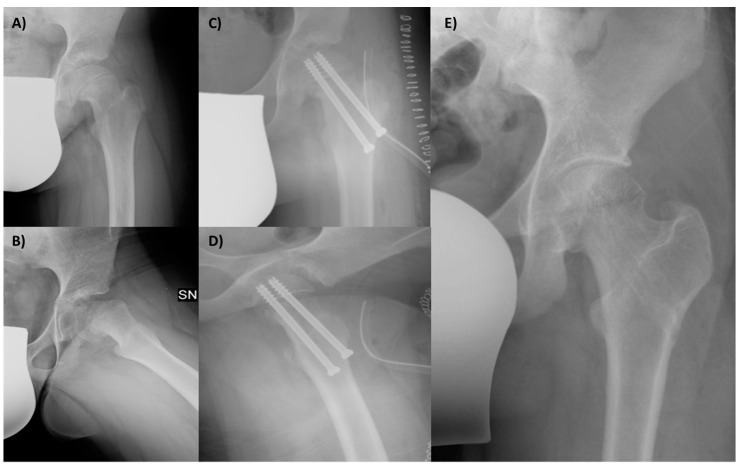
A 12-year-old male patient affected by SCFE, with great deformity of the proximal femur, with a varus (A) and retroverted (B) neck. The femur after the surgery, with the correction in the coronal (C) and sagittal planes (D); the screws in both views are in the bone and not protrude into the joint. The final X-ray follow-up at 8 years shows the outcome of removing screws and the radiological good hip joint status (E).
